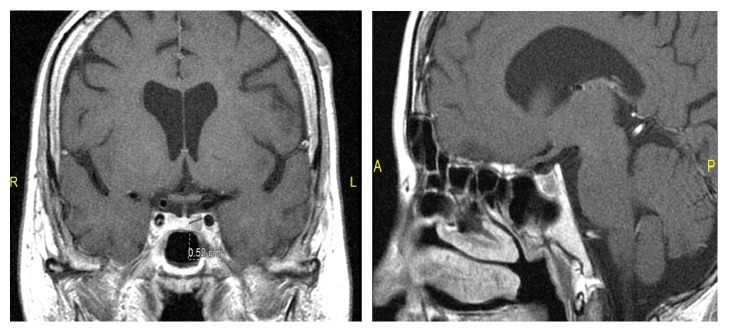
Coronal and sagittal T1-weighted image, showing a 5 mm microadenoma at the left side of pituitary gland.
